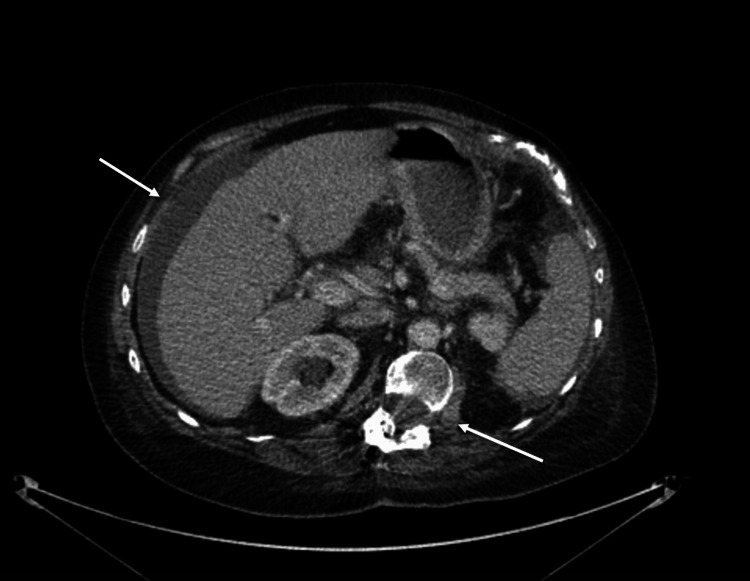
CT abdomen and pelvis from case one demonstrating abdominal ascites with retroperitoneal inflammation.
